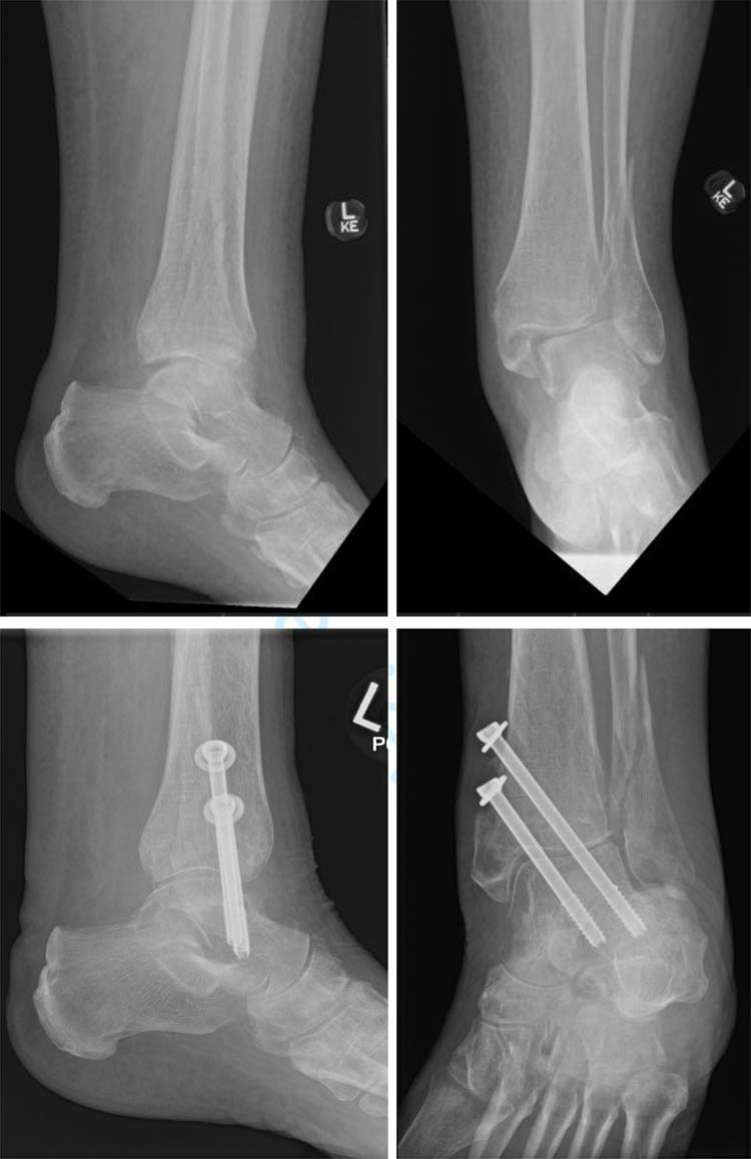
Top—Bimalleolar ankle fracture with talar shift. Bottom—Radiographs at 3 months follow-up showing maintained tibiotalar alignment.
